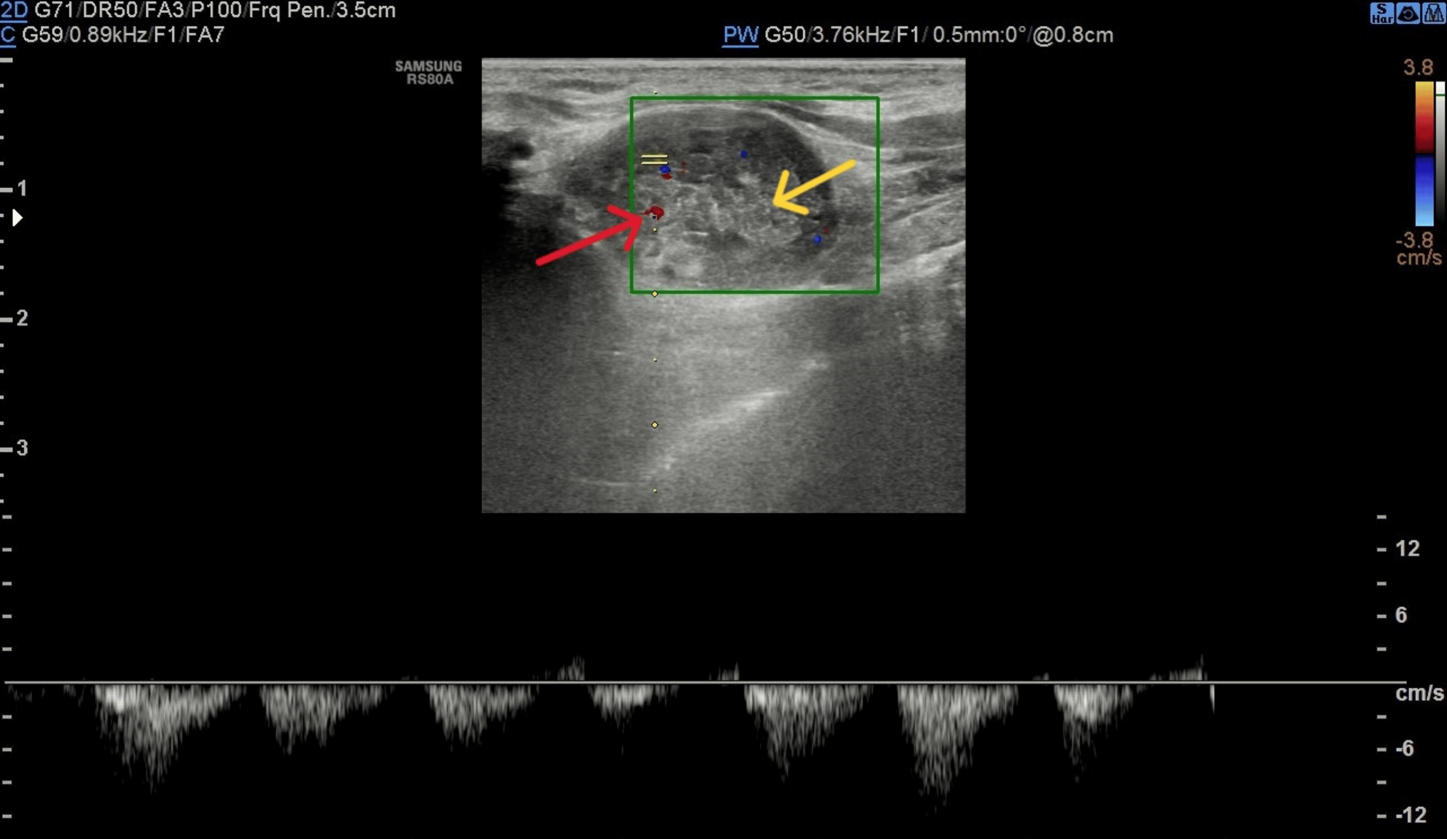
Image from Doppler ultrasonography: apart from arterial flow (red arrow) in the lesion, solid elements with heterogeneous echostructure (yellow arrow) are presented
